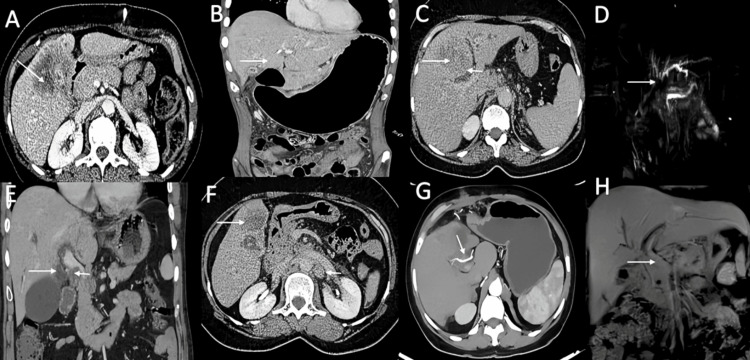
CECT findings in GBC Axial and coronal CECT images (A-H) demonstrate a heterogeneously enhancing gallbladder mass (arrows) with direct invasion of adjacent hepatic segments IVb and V, loss of the fat plane between the gallbladder and liver, and local hepatic infiltration. Associated findings include gallbladder wall thickening, regional lymphadenopathy, and features suggestive of advanced-stage disease. These radiological findings are consistent with locally advanced GBC. GBC: gallbladder carcinoma, CECT: contrast-enhanced computed tomography
